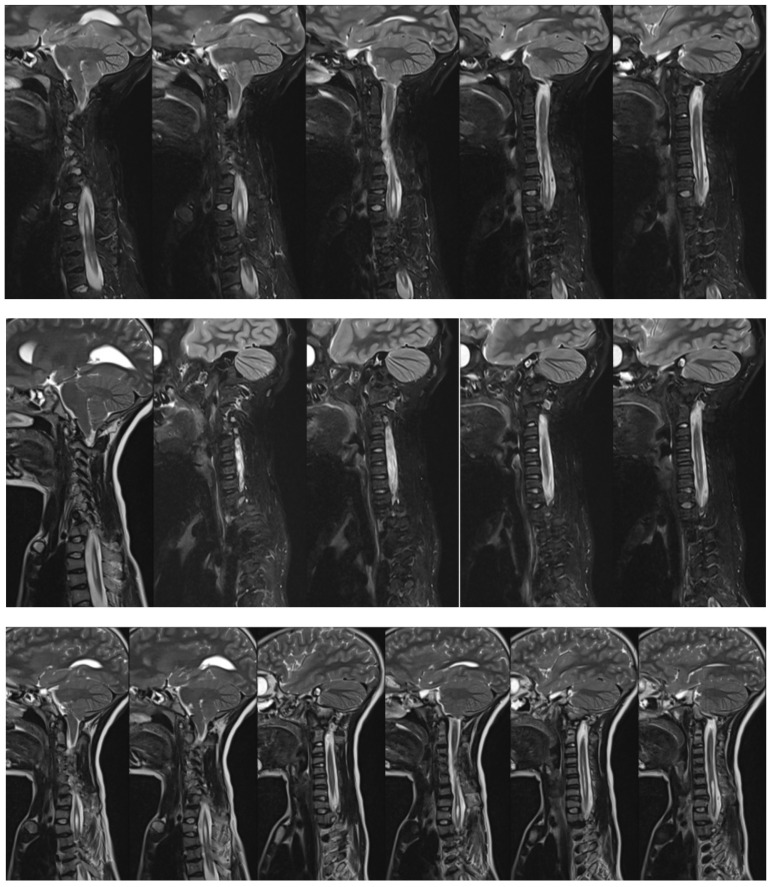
MRI screening of 14-year-old boy with Costello syndrome before surgical treatment of severe scoliosis showed Chiari II and syrinx.
